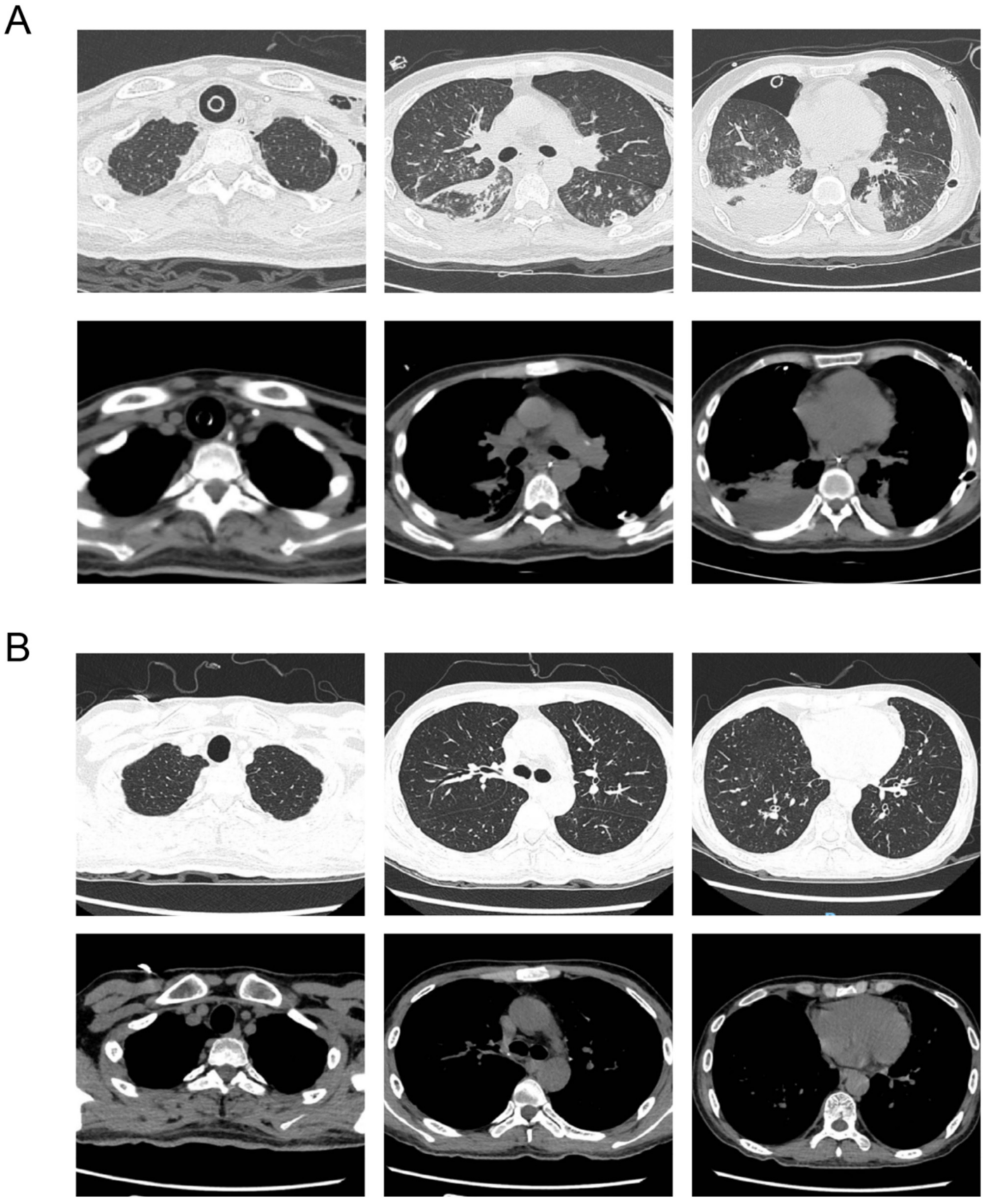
Chest CT evolution after lung transplantation. (A) Chest CT obtained one week after lung transplantation shows clear donor lungs with no signs of infection or rejection. (B) Follow-up CT at 1 month post-transplant demonstrates stable graft appearance and improved aeration, with no radiologic complications.
